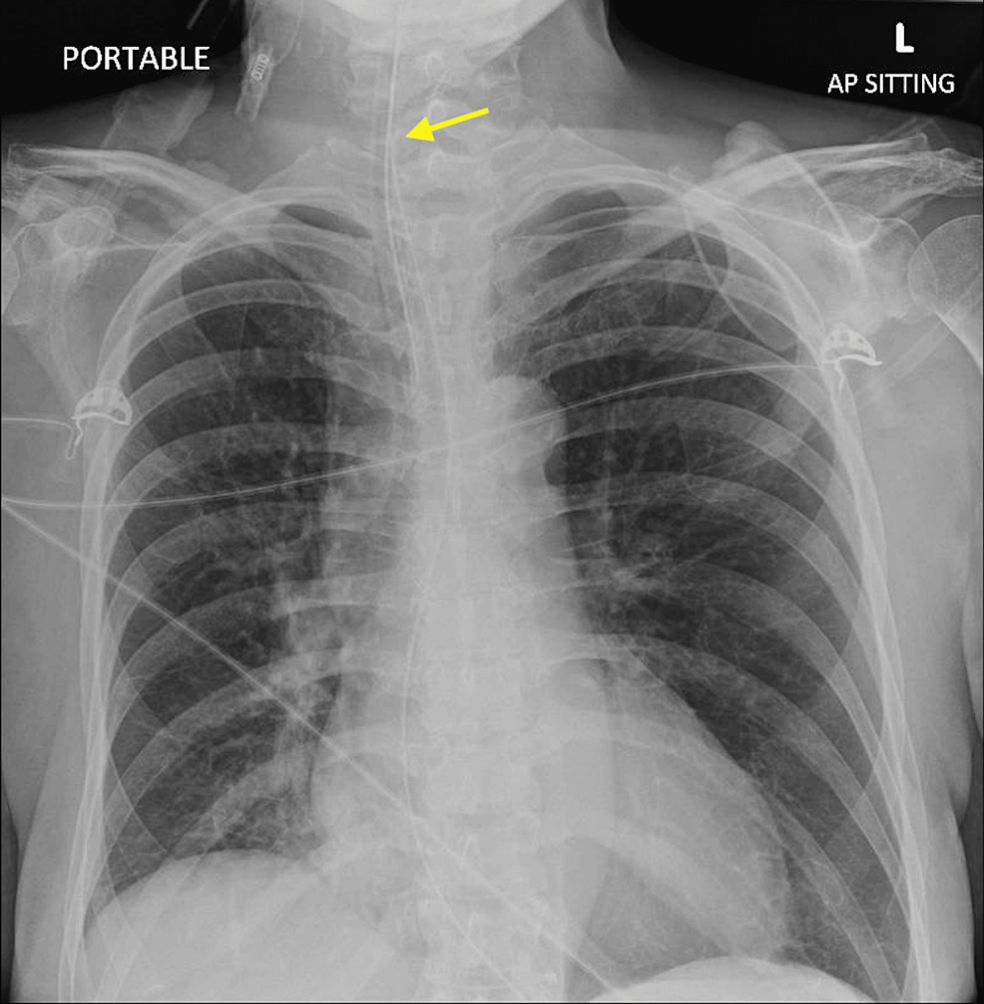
Chest radiograph image showing the positioning of endotracheal and nasogastric feeding tubes post collapse.
